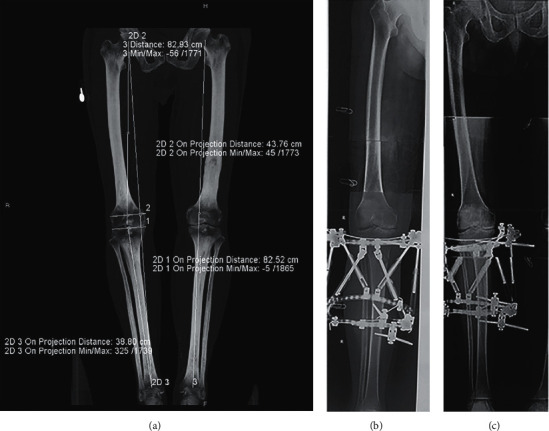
(a) 53-year-old male suffered with knee osteoarthritis combined with knee varus (left: preoperative X-ray shows hyperosteogeny at the edge of the right knee joint, tibial eminence, upper and lower margin of the patella, hardening of joint surface, and the narrowing of joint spaces inside the knee). TSF-assisted OWHTO surgery was conducted on the right knee (b). One month after surgery, the full-length X-ray of the double lower limb shows that correction of the right lower limb assignment is fair (c).
